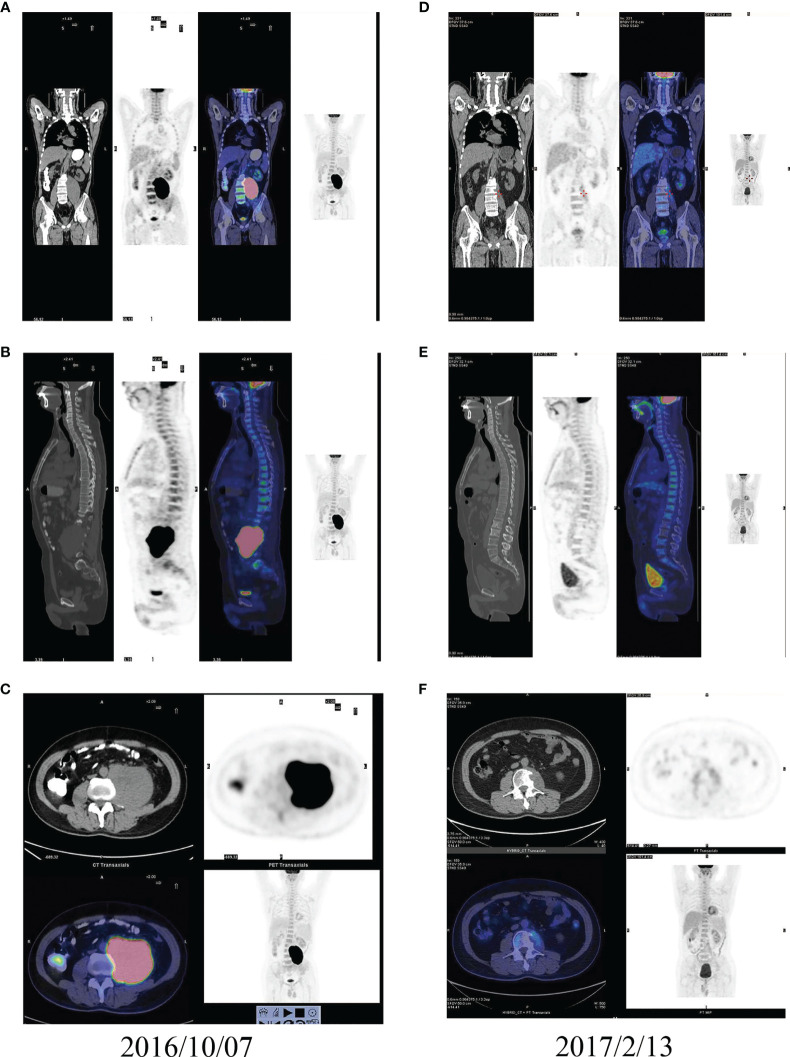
PET-CT comparison of a typical patient with a large retroperitoneal UPS who achieved CR before and after treatment with Endostar combined with AI. (A) Coronal PET-CT image before treatment. (B) Sagittal PET-CT image before treatment. (C) Cross-sectional PET-CT image before treatment. (D) Coronal PET-CT image after treatment. (E) Sagittal PET-CT image after treatment. (F) Cross-sectional PET-CT image after treatment.
